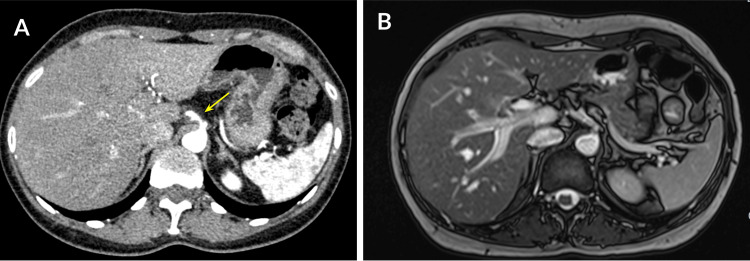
Upper abdominal IV contrast CT and an MRI with IV contrast with gadolinium. A) Arterial phase of IV contrast CT showing a filling defect in the dorsal region of the celiac trunk (yellow arrow). B) Contrast-enhanced T2 MRI demonstrating stenosis that caused a reduction in arterial blood flow of up to 50% in a 5.6 mm segment with a deflection angle of the celiac trunk of 79°. MRI, magnetic resonance imaging; IV, intravenous; CT, computed tomography
